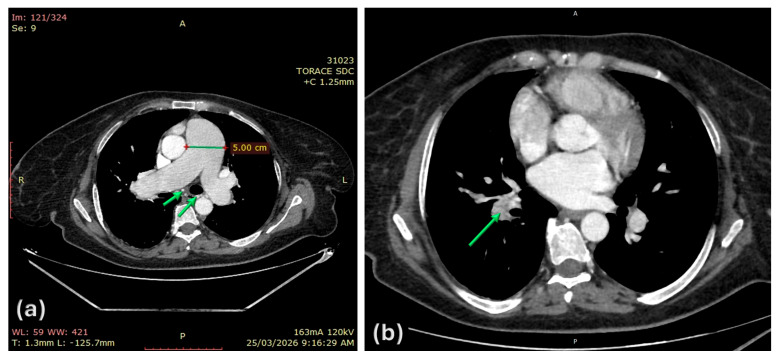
The chest CT angiography performed in 2026 shows progressive dimensional increase in the caliber of the pulmonary artery trunk (a) and almost complete chronic thrombosis of the left inferior lobar pulmonary artery (b), with MAPCA-type aorto-pulmonary arterial collateral circulation (arrows in (a)).
